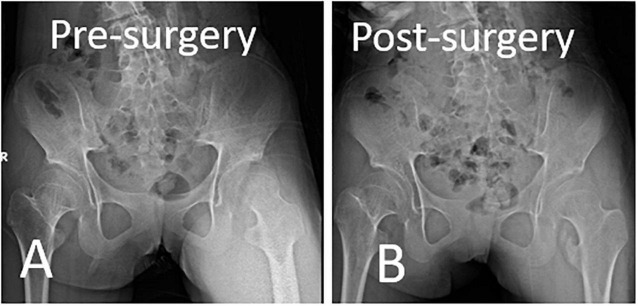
Immediate postoperative anteroposterior pelvis x-ray showed tailored removal of ossification mass according to the preoperative plan. (A) Preoperative X-ray. (B) Immediate postoperative X-ray.
